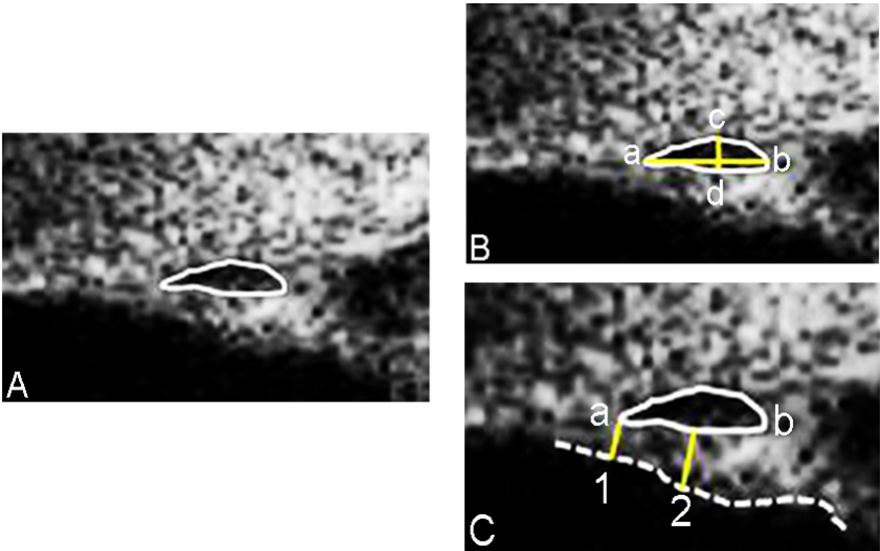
Example of Schlemm’s Canal and Trabecular Meshwork Measurements Made Using the iUltrasound Imaging System. The black oval space shows Schelmm’s canal (SC). The meridional diameter of SC was measured from the anterior (a) to the posterior (b) end point of SC. To measure the coronal diameter of SC, we drew a vertical line across the canal to get two intersection points (c and d). The maximum distance between c and d was taken as the coronal diameter of SC. Lines 1, 2 indicated where trabecular meshwork thickness was measured and the dotted line shows the meshwork inner layer.
